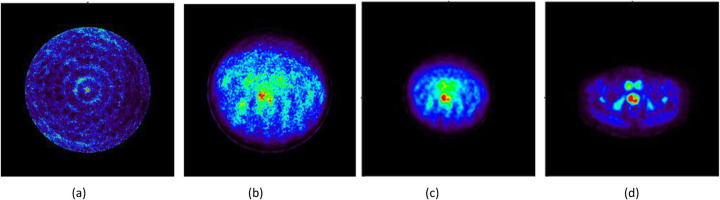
Image reconstructions using the prostate PET prototype of the patient data before normalization (a), with only normalization (b), also including CT-based attenuation (c). Image of one bed position with the Gemini-TF is depicted in d
